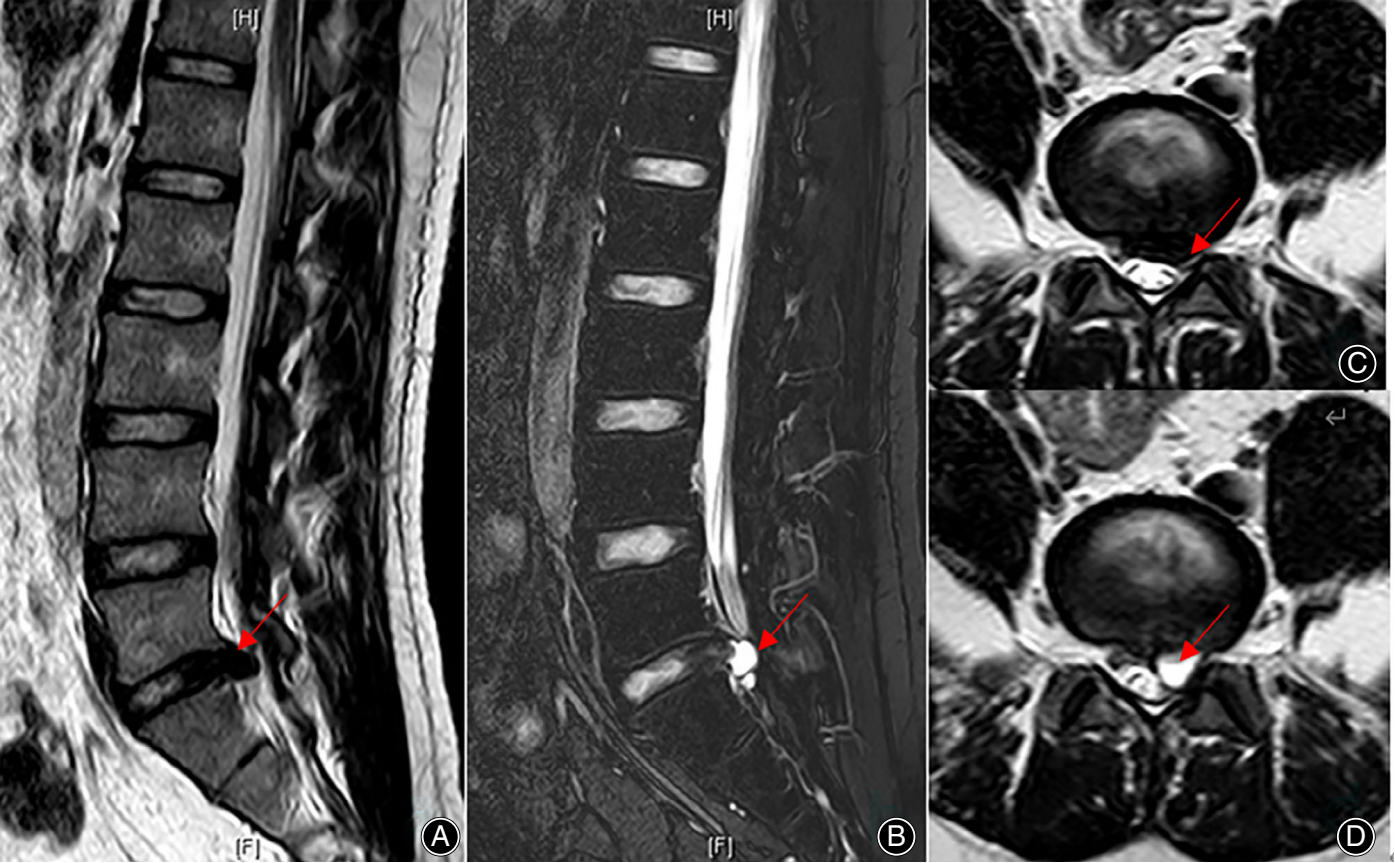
Lumbar MRI: disc degeneration (Pfirrmann Grade III) and PDP. A\C: disc degeneration before lumbar discectomy, B\D: PDP (L5/S1 left).
